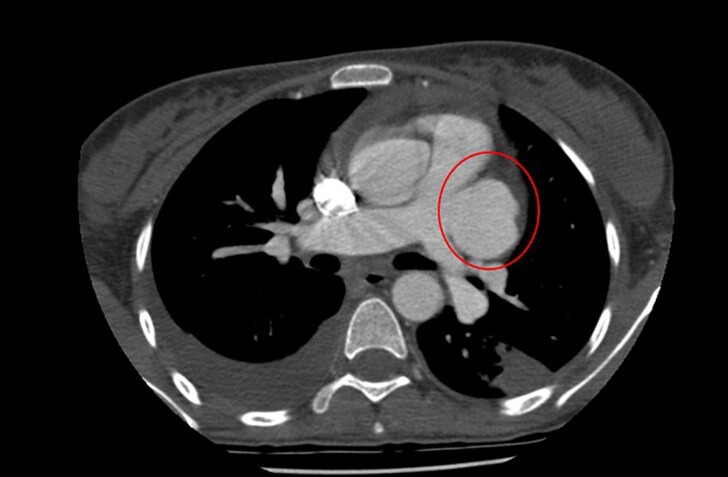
CT scan showing aneurysms on the trunk of the pulmonary artery.
